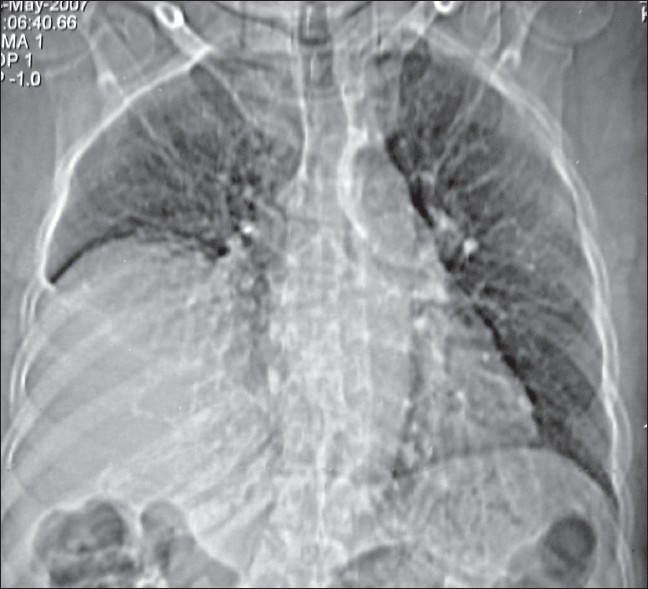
CT scan of the chest showing complete eventration of right dome of diaphragm
